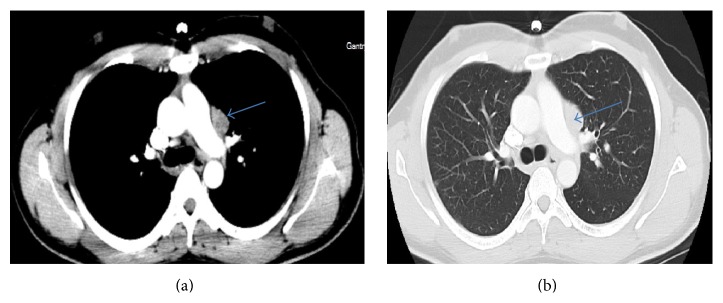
Axial CT scans of the chest ((a) mediastinal window; (b) lung window) showing large mediastinal lymph node with normal lung parenchyma before anti-TB therapy.
